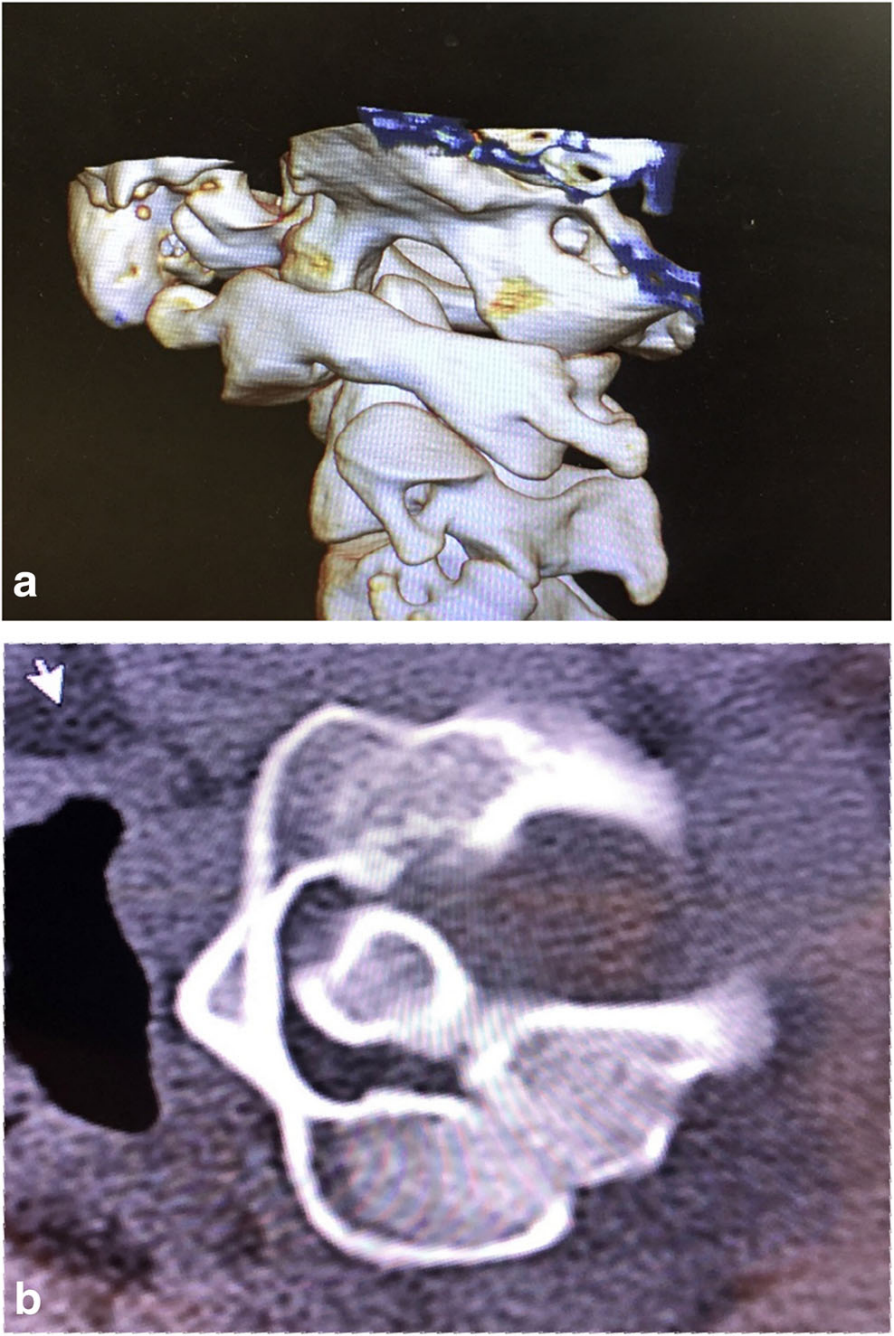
a A 3D CT reconstruction of the cervical spine rotated to the left shows that the left superior facet of C2 is more than 90% exposed, and, therefore, defined as subluxed. b Axial CT of C1 and C2 showing neck rotation to the right. Note the Fielding type 1 subluxation, with anterior subluxation of the left C1 facet (anterior to the left C2 facet), while the atlanto-dental interval—between the anterior tubercle and the odontoid—remains normal
